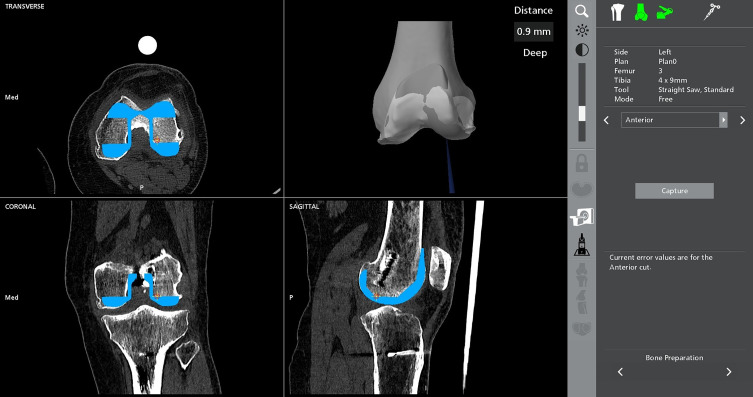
Preoperative planning of the femoral component and boxing cut using an image-based robotic system. CT-based imaging was utilized to precisely position the femoral implant while ensuring the cutting box aligned with the head of the femoral fixation screw. The robotic system provided detailed visualizations in transverse, coronal, and sagittal planes.
